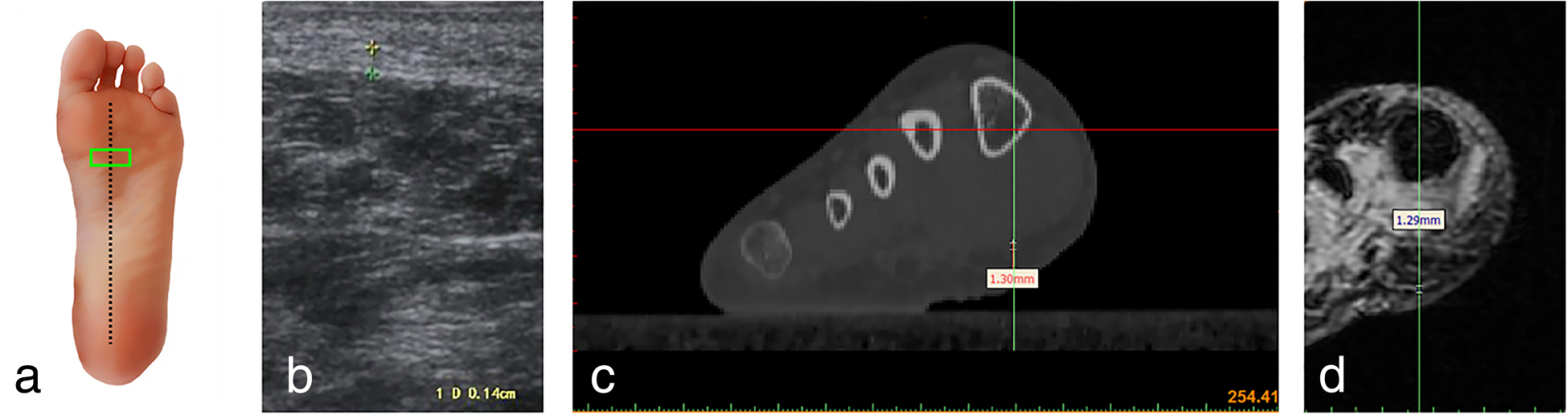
Ultrasound, CT and MRI measurements of the thickness of the plantar fascia between the first and second toes (transverse section) a Ultrasonic probe is placed (green panel). b Ultrasonic measurement. c Measuring the thickness of the plantar fascia reconstruction CT images. d Measuring the thickness of the plantar fascia reconstruction MRI images
